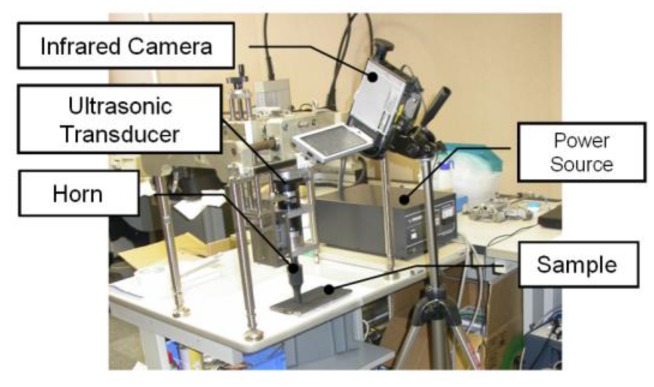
Experimental set-up of ultrasonic stimulated thermography, from [98].
